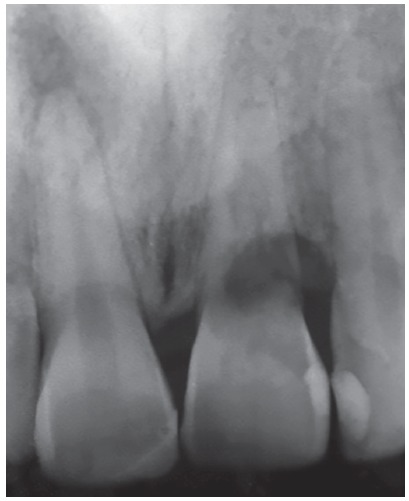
Diagnostic radiography of the maxillary left central incisor with replacement resorption induced by dental trauma, whose prognosis is the inevitable tooth loss. The right central incisor also suffered trauma, but without progression to dentoalveolar ankylosis, and evolved to aseptic pulpal necrosis.
